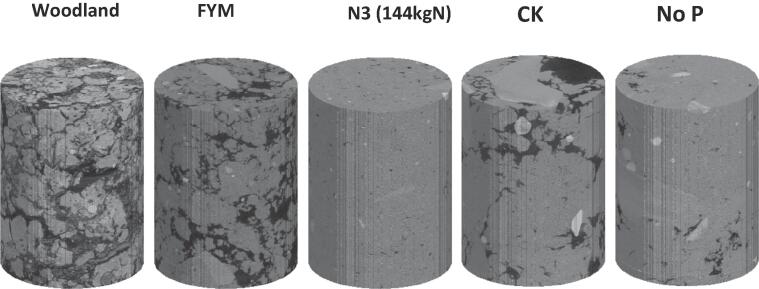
X-ray CT images of cores (40 μm resolution) taken from plots under different fertilizations and the woodland.
